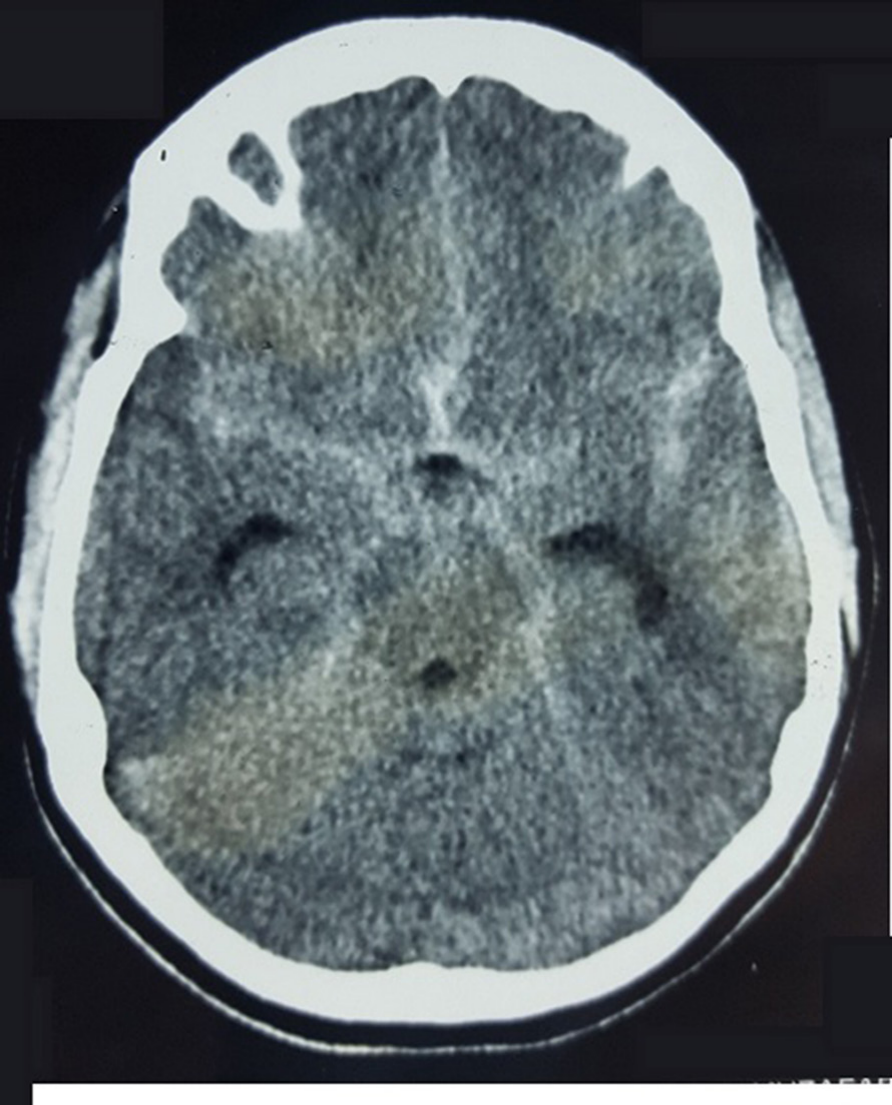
Initial axial CT scan, demonstrating diffuse subarachnoid haemorrhage and cisternal clot at level of interpeduncular cistern.
